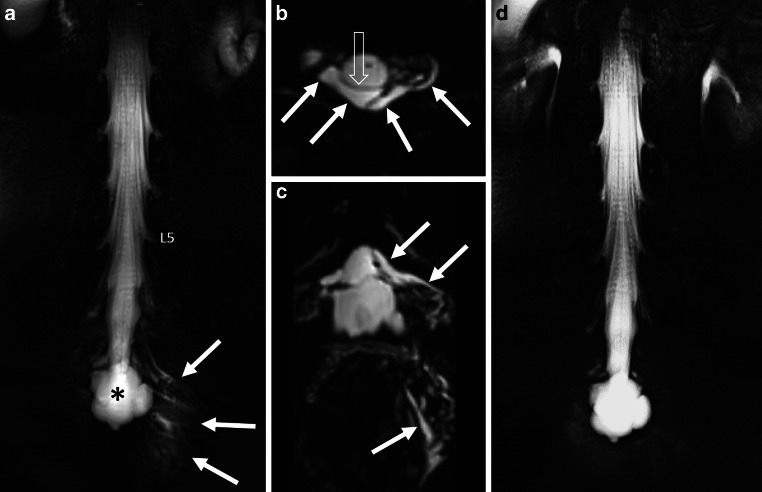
34-year-old woman with a sacral dural leak and SIH score of 7. Spinal longitudinal extradural CSF collection (SLEC) is exclusively visible at the sacral level on coronal 2D heavily T2-weighted MRI (T2-MRM) (*arrows* in a) and on axial and coronal 3D T2-MRM (*arrows* in b, c), representing the sacral origin of leak. The SLEC is well distinguishable from the dura (*open arrow* in b). A large meningeal cyst/dural ectasia is visible on coronal 2D T2-MRM (*asterisk* in a). After lumbar epidural blood patch (EBP) in reversed Trendelenburg position, SLEC completely resolved (d)
